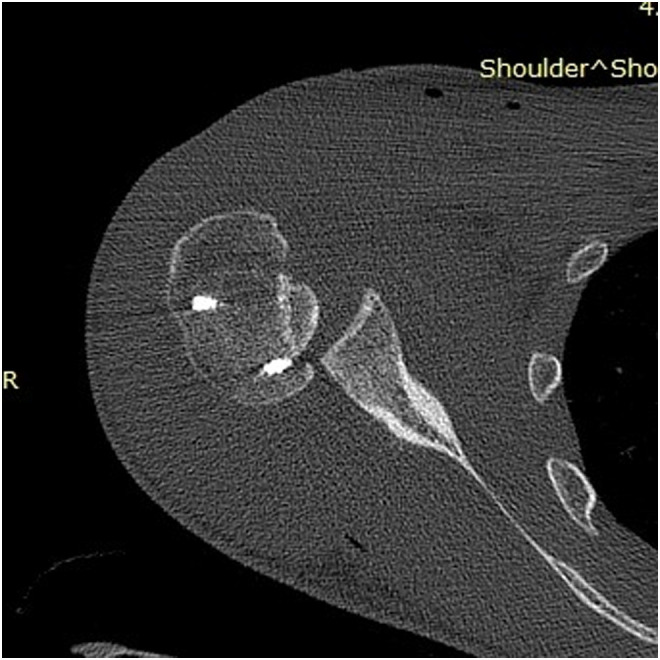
1st patient: Postoperative CT scan confirming the persistence of subluxation between reverse Hill-Sachs lesion and posterior Bankart lesion – after the modified McLaughlin procedure, in which only the humeral head’s side was addressed.
